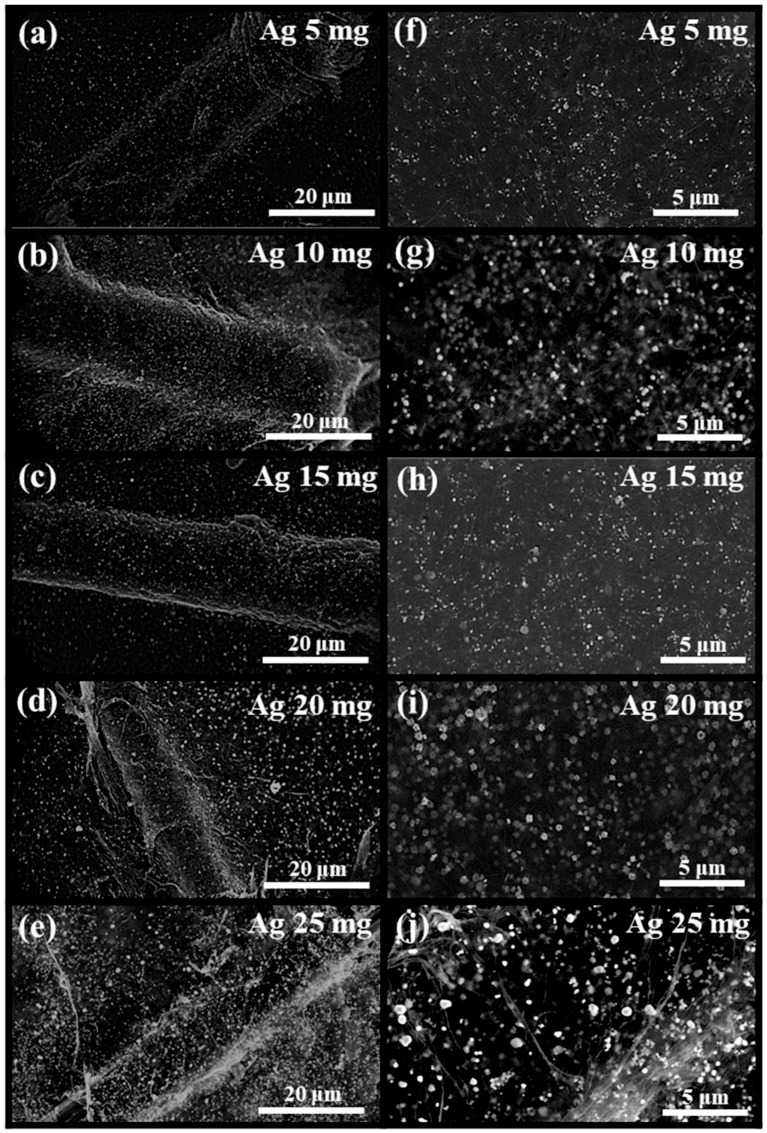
HR-SEM images of the FWCNT-MPCF-Ag hybrid films incorporated with different Ag contents of: (a,f) 5 mg (9.1 wt %), (b,g) 10 mg (16.6 wt %), (c,h) 15 mg (23.0 wt %), (d,i) 20 mg (28.6 wt %), and (e,j) 25 mg (33.3 wt %) (MPCF length: 6 mm; MPCF content: 30 mg; FWCNT content: 20 mg; ultrasonication for 0.5 h). (a–e) are low-magnification images, while (f–j) are high-magnification images.
